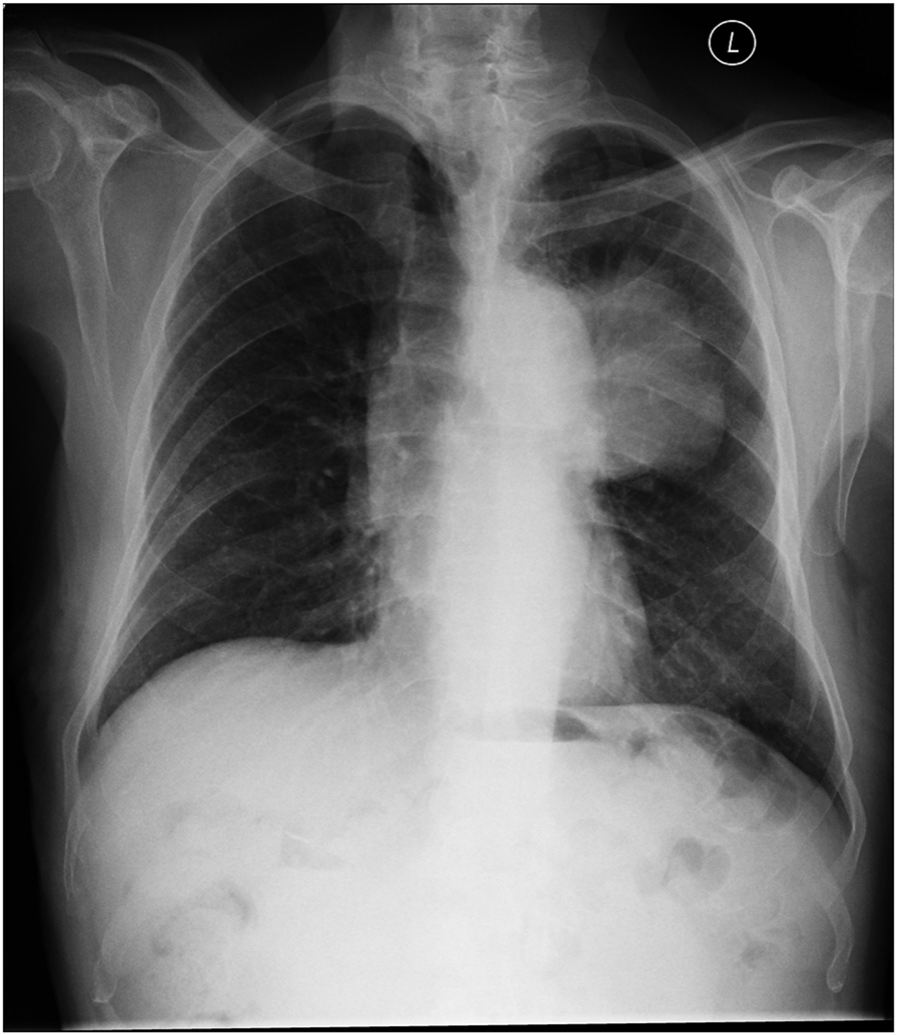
Sagittal head computed tomography slice. This image is a sagittal slice of the head computed tomography performed on the patient. It demonstrates the intracranial metastasis.
